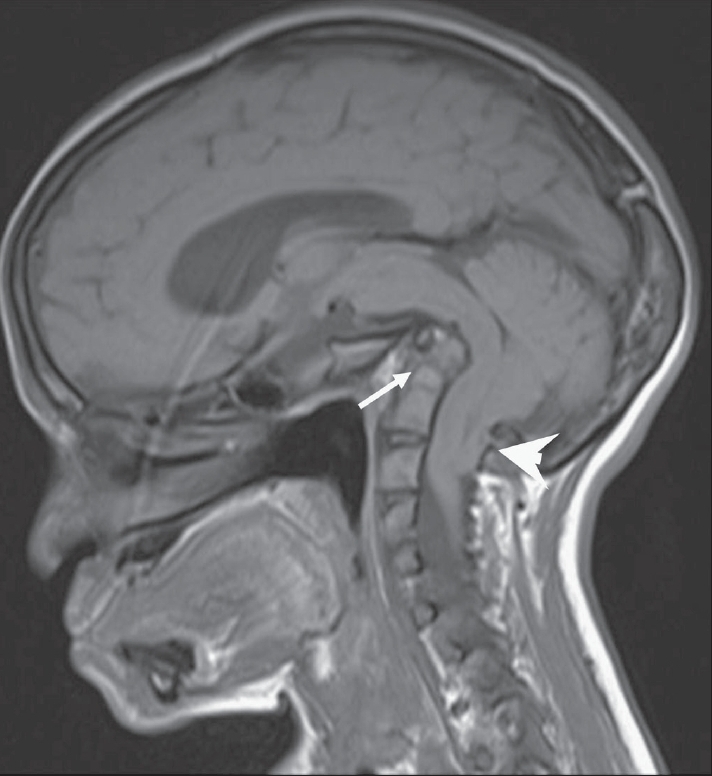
Sagittal T1W MRI shows basilar invagination (arrow) with an Arnold-Chiari I malformation (arrowhead)
